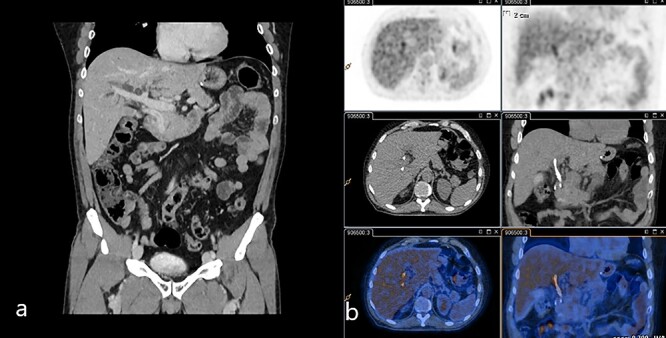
(a) Venous phase of the CT scan showing the dilated biliary tree and the contrast-enhanced thickening of the common bile duct. (b) 18-FDG-PET/CT scan showing the slight uptake of marked glucose along the common bile duct.
